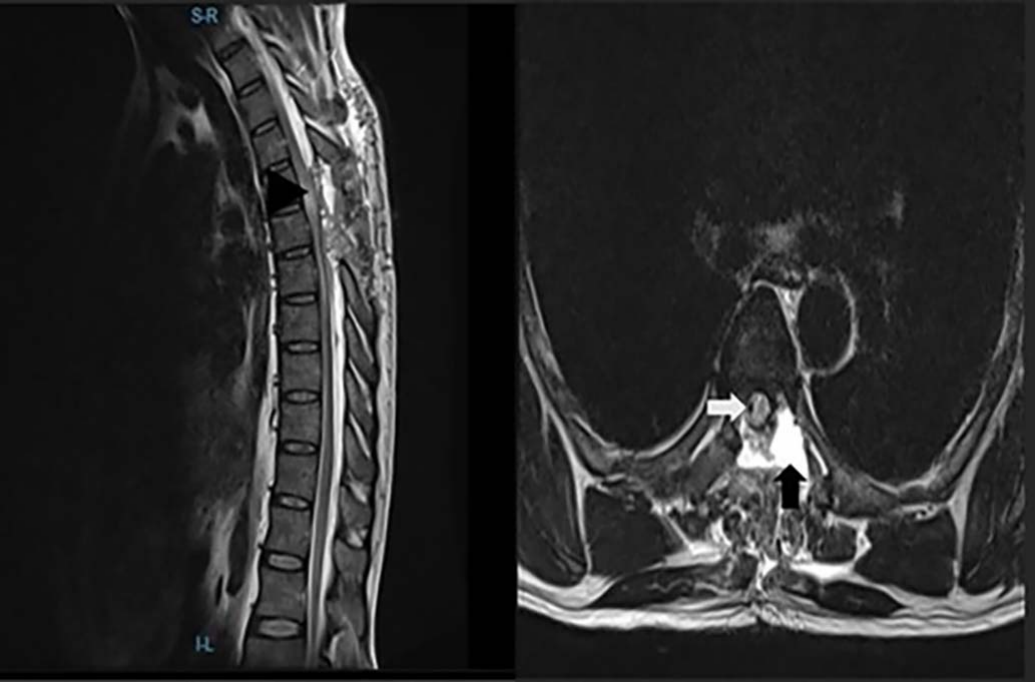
Thoracic spine magnetic resonance imaging sagittal (left) and axial (right) views show complete resection of the arachnoid cyst, cord reexpansion (white arrow), a small extradural cerebrospinal fluid collection (black arrow), and associated cord edema (black arrowhead).
